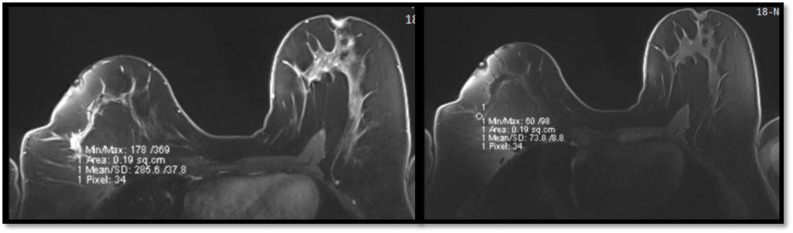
Breast magnetic resonance imaging scans for a 49-year-old patient with an incidental finding.
